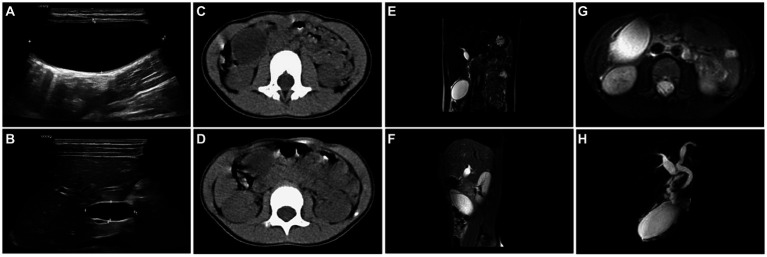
(A) Abdominal ultrasound showed a cystic area of 7.2 cm × 3.1 cm × 3.8 cm in the right upper abdomen, with clear boundaries and sound transmission. (B) Abdominal ultrasound showed a cystic area of 2.7 cm × 1.1 cm × 1.4 cm at the gallbladder fossa, with clear boundaries and sound transmission. (C,D) Abdominal CT showed an oval low-density shadow, about 65 mm × 26 mm × 30 mm in size, with a clear boundary in the right upper abdomen (lower edge of the liver). The gallbladder was not clear, and cystic shadow was seen in the gallbladder fossa area, and the common bile duct was slightly dilated. (E) Coronal T2-W MRI showed that there was no normal gallbladder and cystic duct in the gallbladder fossa area, an ovoid cystic high signal was seen in the lower layer of the gallbladder fossa, and a tortuous tubular structure was seen in the anterior superior layer to the direction of the gallbladder fossa. (F) Sagittal T2-W MRI showed that there was no normal gallbladder and cystic duct in the gallbladder fossa area, and the oval cystic high signal was observed at the level below the gallbladder fossa. (G) Horizontal T2-W MRI showed an ovoid cystic high signal (52 mm × 30 mm) below the gallbladder fossa, and the fluid signal was seen around the cystic structure. (H) MRCP showed a tortuous tubular structure above the gallbladder, which was highly suspected of gallbladder torsion.
